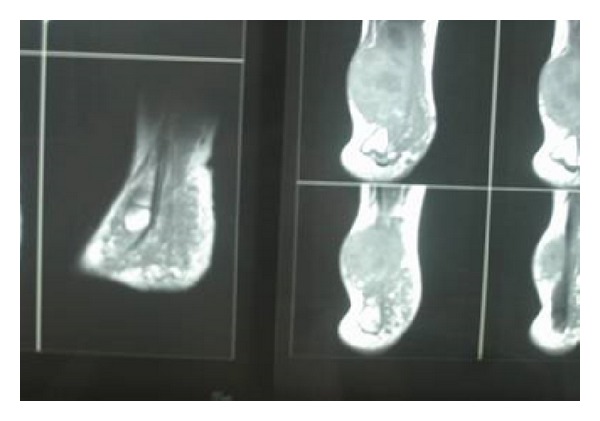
MR images showed the presence of a huge mass of the right calf and foot.
